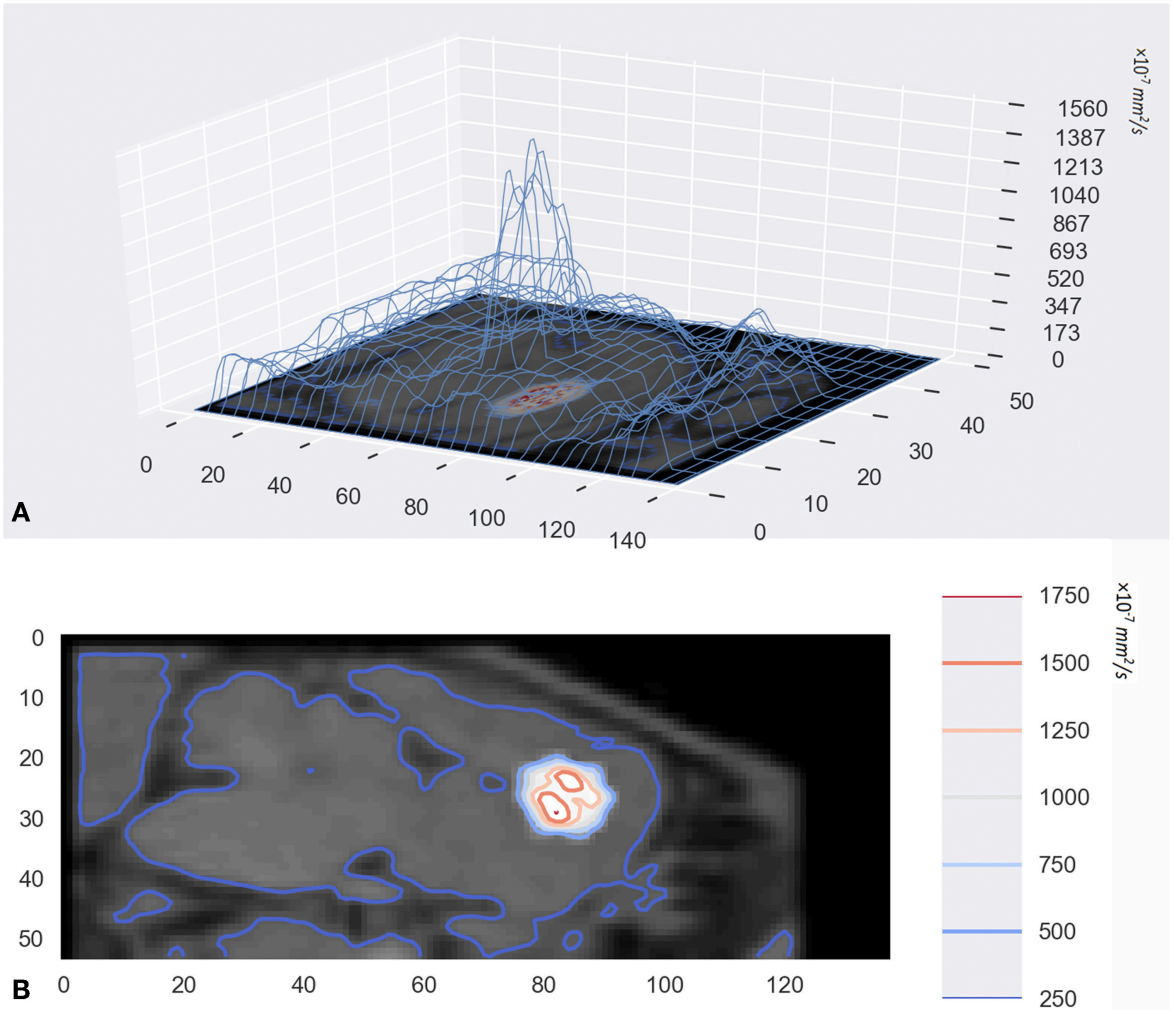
(A) was the 3D wireframe and the height of the contour line represents the level of D. Panel (B) was a contour map superimposed on the original MR image. They were drawn with a Python package named Matplotlib.
